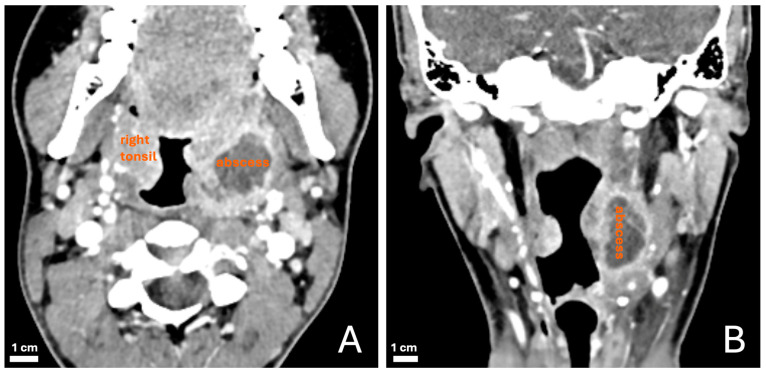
Radiological criteria used for peritonsillar abscess (PTA). Contrast-enhanced axial (A) and coronal (B) CT slices. Here, PTA is defined by fluid collection in the left peritonsillar space with serpiginous, irregular, and thick peripheral rim enhancement.
